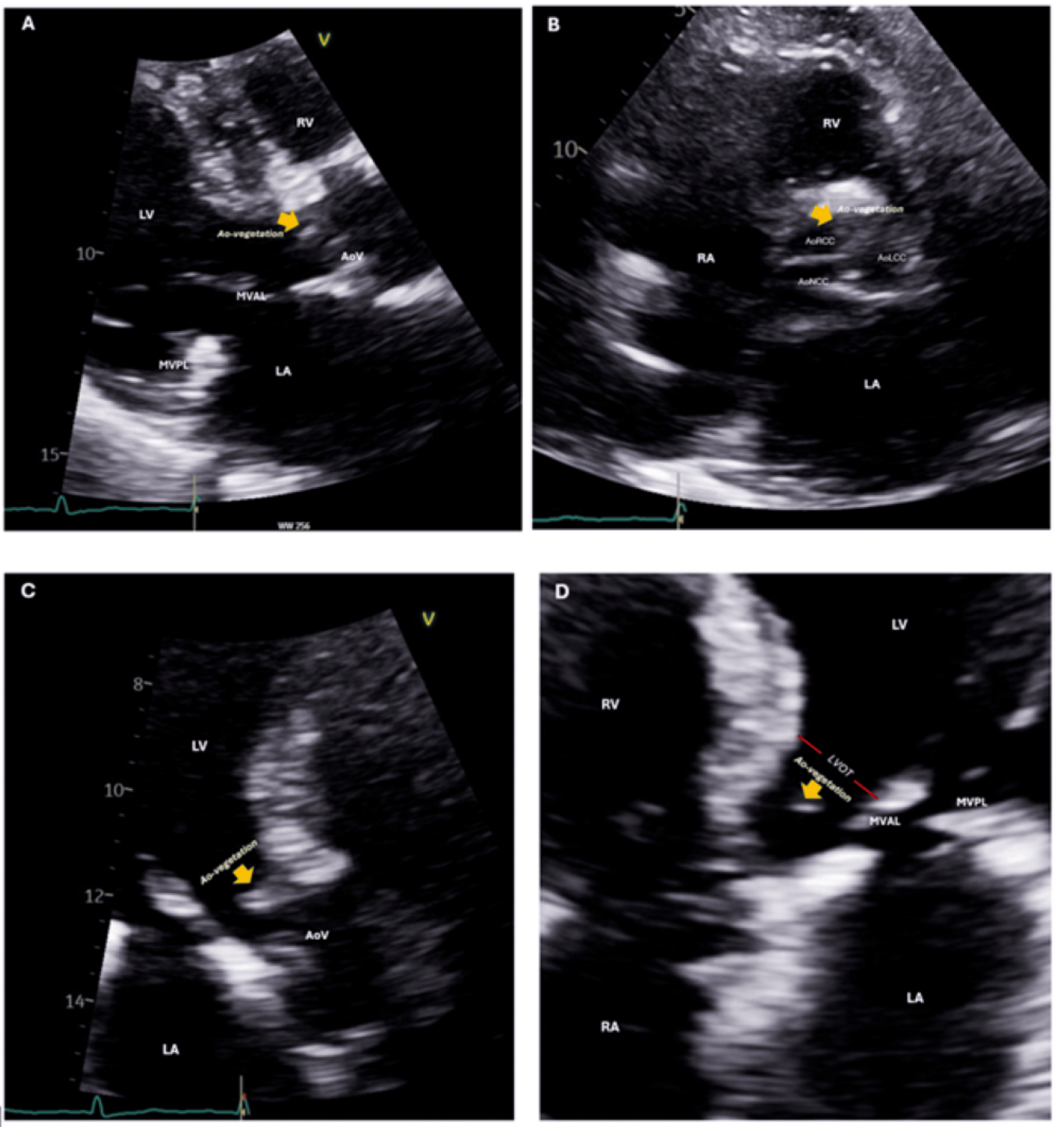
Two-dimensional transthoracic echocardiography (Panel A: parasternal long-axis view, Panel B: parasternal short-axis view, Panel C: apical three-chamber view, and Panel D: apical five-chamber view) demonstrates a trileaflet aortic valve with leaflet thickening but preserved systolic excursion. Notably, a sclerotic, prolapsing structure is visualized between the right and left coronary cusps, raising suspicion for valvular vegetation (yellow arrow). LA: left atrium RA: right atrium LV: left ventricle MVAL: mitral valve anterior leaflet MVPL: mitral valve posterior leaflet LVOT: left ventricular outflow tract
